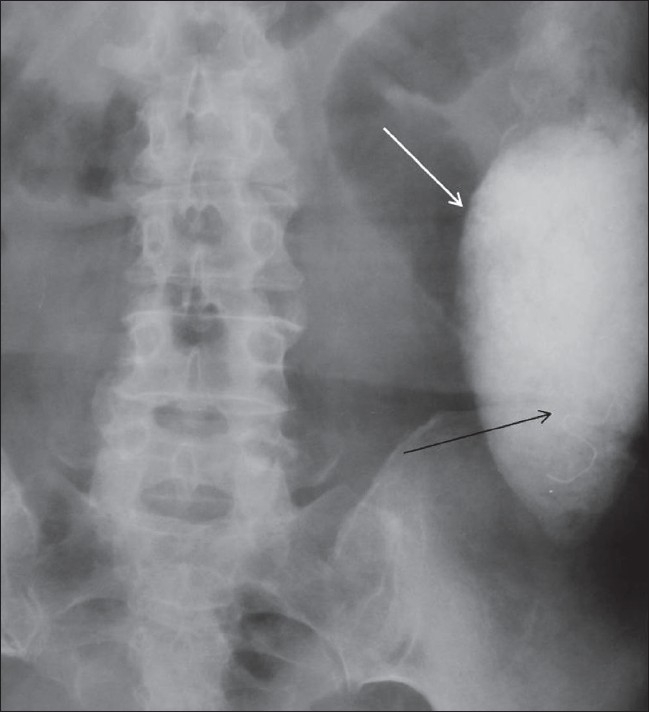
Plain radiograph obtained 6 h after barium enema shows the mass opacified with barium (white arrow); the marker has migrated inferiorly towards the left lower quadrant (black arrow)
